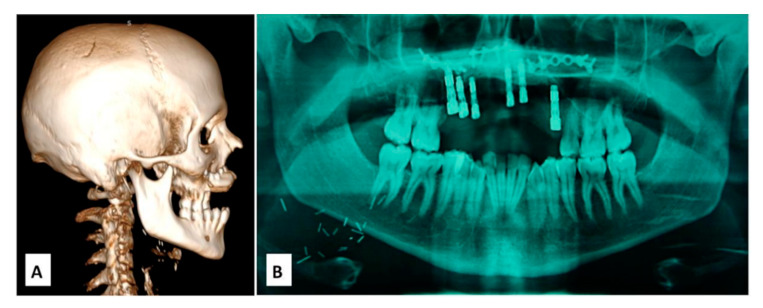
(A) CT (Computed Tomography) scan 3D before prosthetic rehabilitation; (B) dental panoramic radiograph after placement of dental implant.
